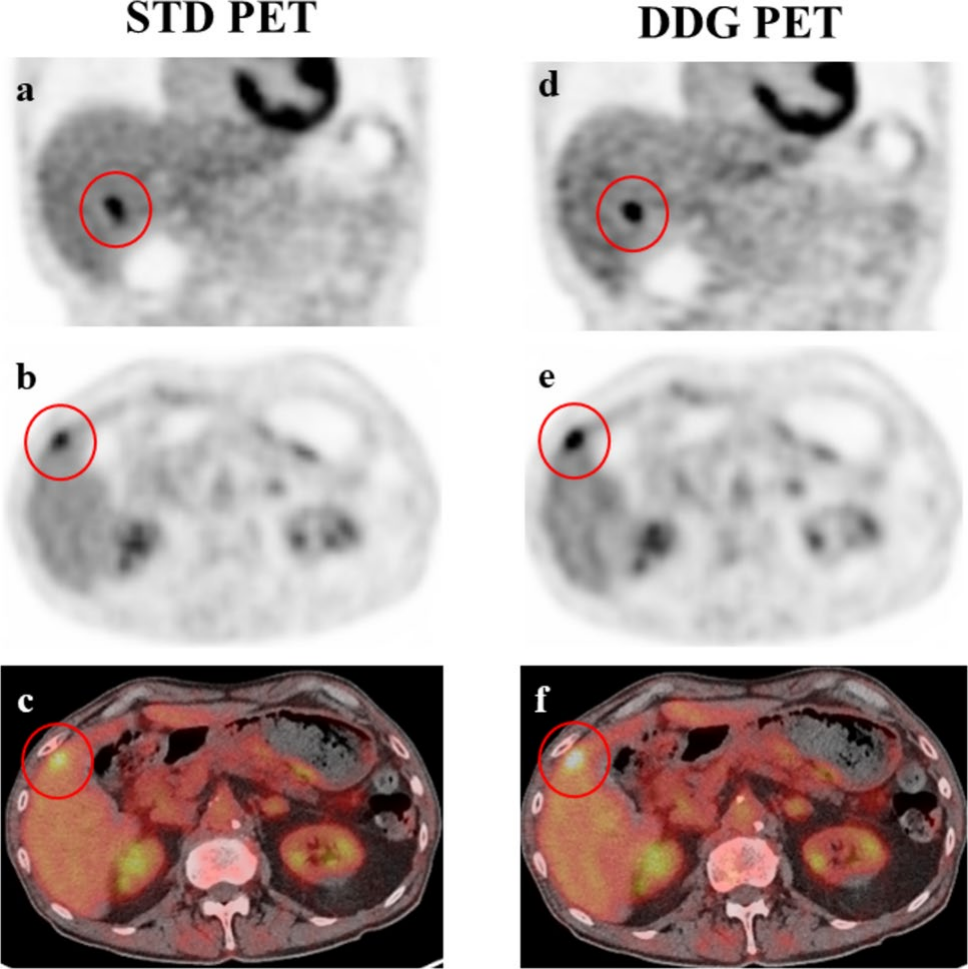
Liver cancer. The liver cancer lesion was visualized with less blurring in DDG than in STD FDG PET. SUVmax and MTV in DDG (9.2 and 2.3 ml) were higher and lower, respectively, than those (5.7 and 5.9 ml) in STD PET. *STD*: standard free-breathing, *DDG*: data-driven respiratory gating. a, d: Coronal FDG PET image, b, e: transaxial FDG PET image, c, f: fused FDG PET/CT image
